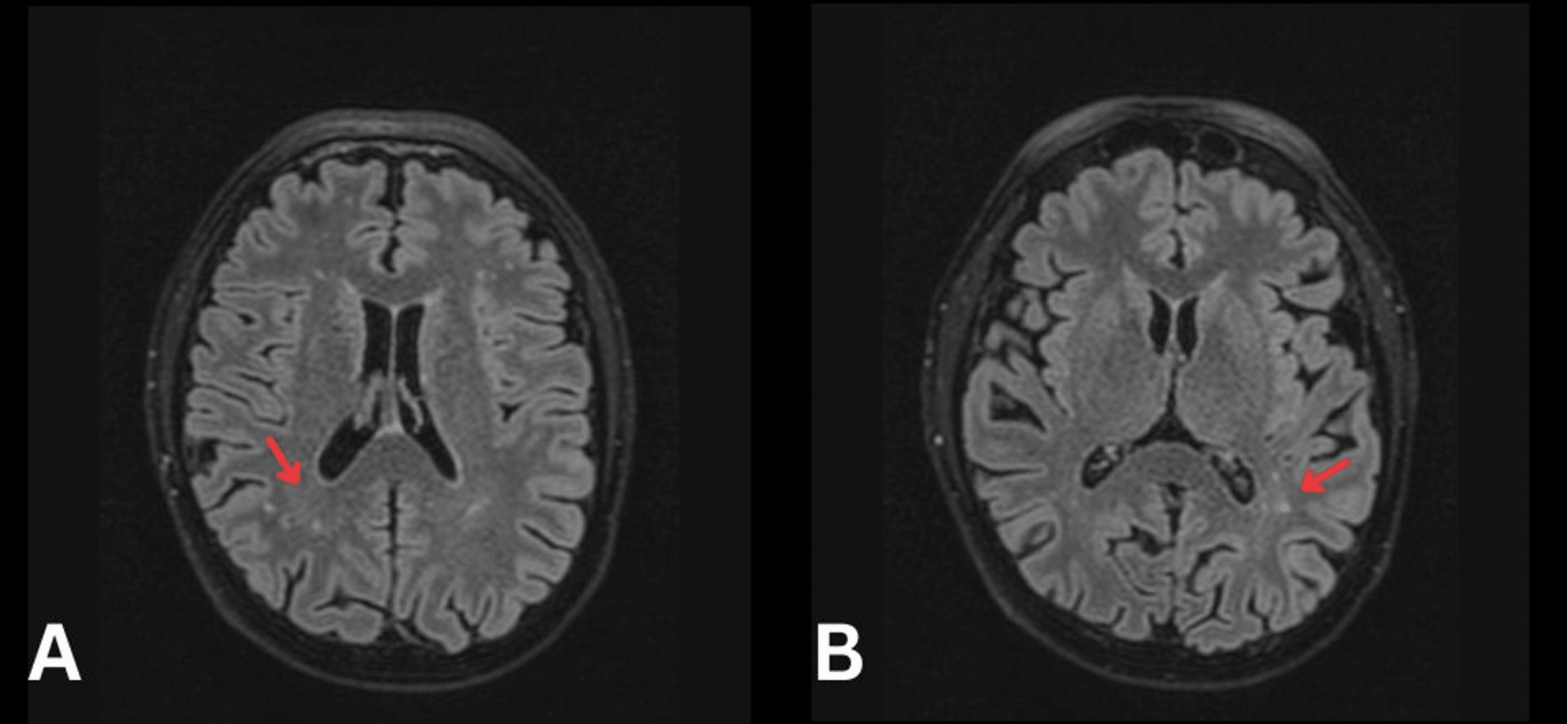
MRI brain T2-weighted imaging A. Right occipital increased signal intensity representing infarct (arrow); B. Left occipital increased signal intensity representing infarct (arrow)
